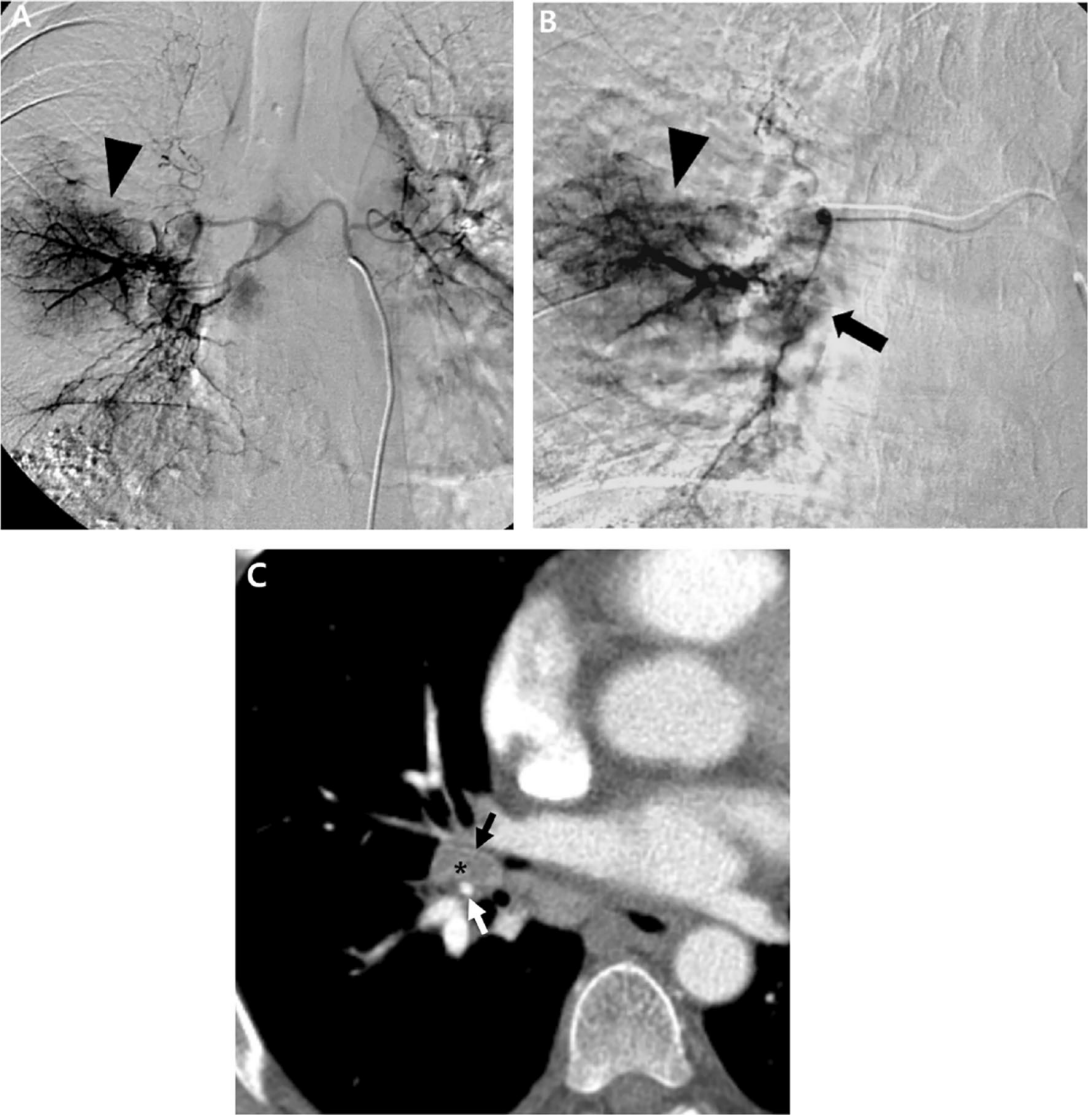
A 56-year-old man with pulmonary metastasis from HCC who presented with a moderate amount of hemoptysis. (A) Selective common bronchial trunk arteriography using a 5-F catheter shows multiple mediastinal tumor blushes and an extensive bronchopulmonary shunt (black arrowhead) in the right middle lobe. (B) Selective left upper bronchial arteriography using a 2.2-F microcatheter shows a bronchopulmonary shunt (black arrow head) and a metastatic lesion responsible for the tumor blush (black arrow). (C) Axial CT image before embolization shows the bronchial artery (black arrow) and pulmonary artery (white arrow) encased by an interlobar metastatic lymph node (black asterisk) between the right middle lobe and right lower lobe bronchus. This finding corresponds with the angiographic finding of a bronchopulmonary shunt.
